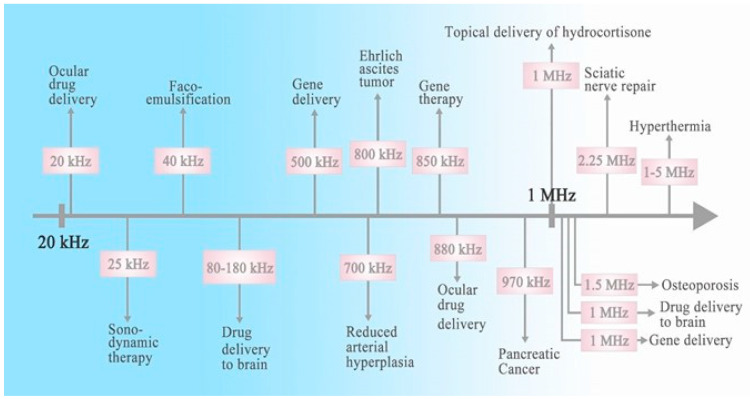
A summary of biomedical applications of different frequencies of ultrasound.
